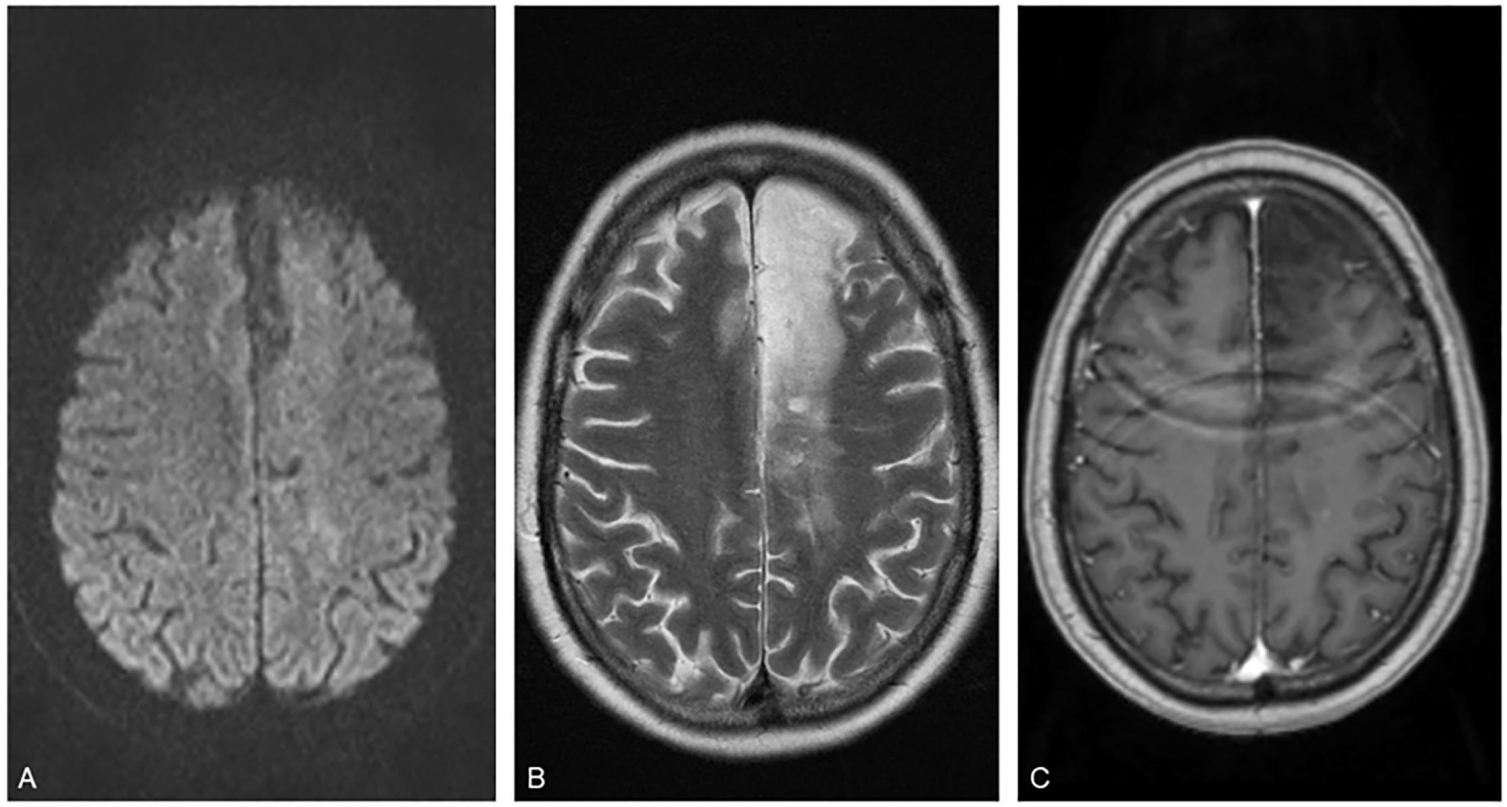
Last follow up. Follow up magnetic resonance imaging (MRI) findings 3 months after latest discharge [(A) Diffusion-weighted imaging, (B) T2 weighted imaging, and (C) contrast enhanced T1 imaging].
